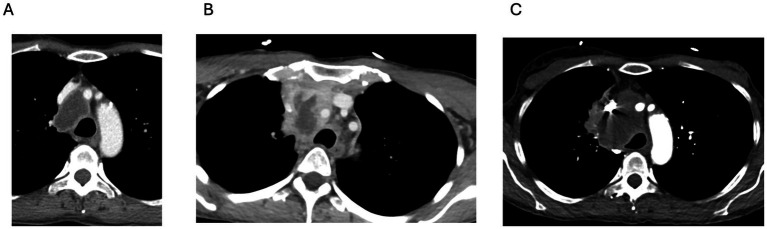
Progressive enlargement of an anterior mediastinal mass over time. (A) CT scan of the chest at presentation showing a 4.0 × 2.4 cm centrally necrotic mass in the middle mediastinum with smooth peripheral enhancement and associated SVC involvement and thrombus. (B) CT scan of the chest taken approximately 3 weeks later, demonstrating interval enlargement of the lesion with increased peripheral enhancement and a new extension into the anterior mediastinum, involving the left brachiocephalic vein. (C) CT scan of the chest taken approximately 8 weeks after the initial presentation, showing further progression with an increase in size to 6.3 × 3.7 cm and possible adjacent lung involvement.
